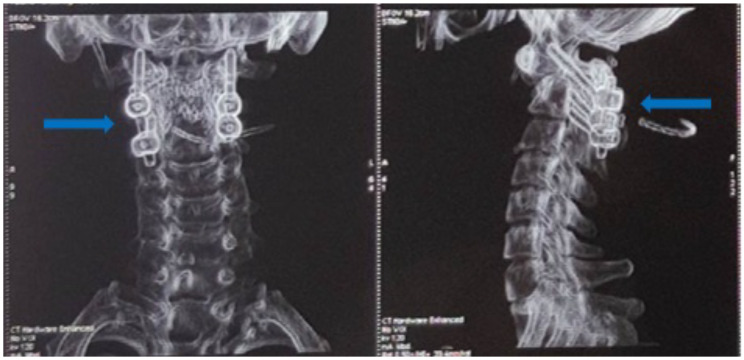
CT-scan postoperative of the cervical spine coronal-sagittal showed the reduction of C1-C2 joint.
